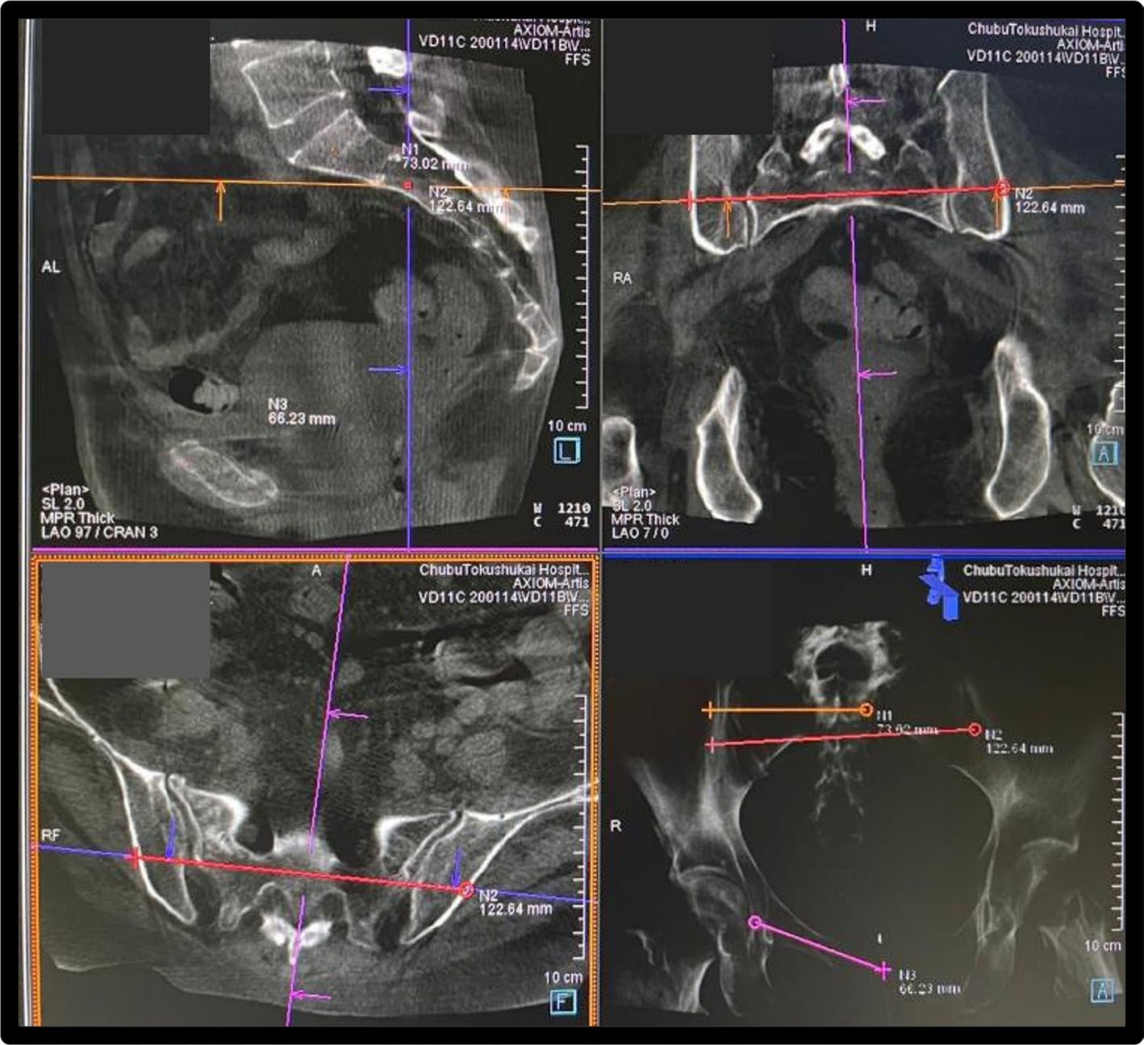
3D preoperative planning. Large field of view and high-quality images were acquired using C-arm CBCT. Data were reconstructed on a workstation. The entry point of the screw trajectory into the cortical bone was indicated using a cross (×) and the end point of the screw tip was indicated with a circle (○).The screw trajectory planning and overlay software used was an inbuilt technology for needle biopsies
